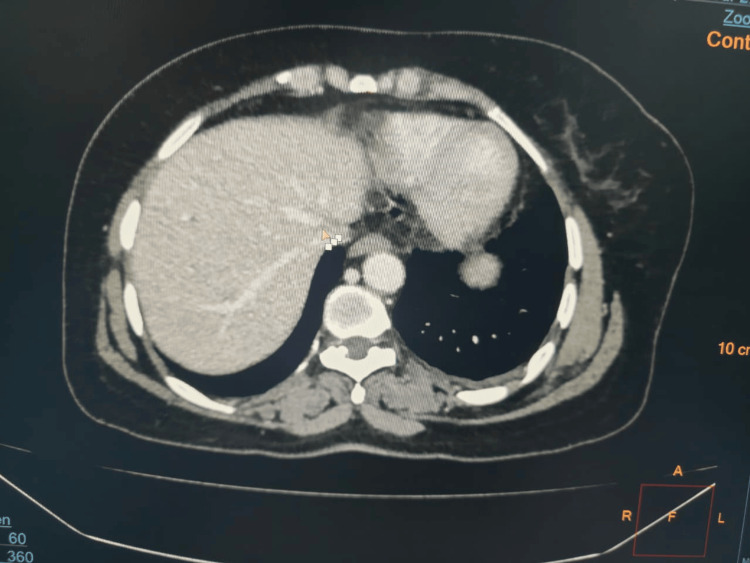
CT image showing an opacification defect of the portal vein CT: computed tomography
